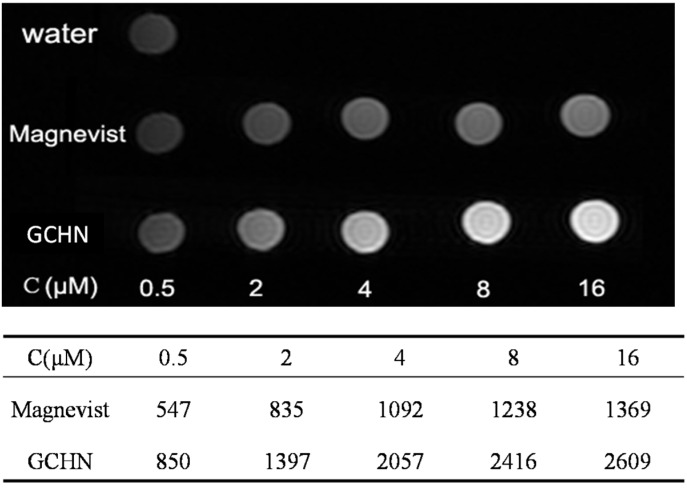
MRI images and the signal intensity *in vitro* of Magnevist and GCHN.
